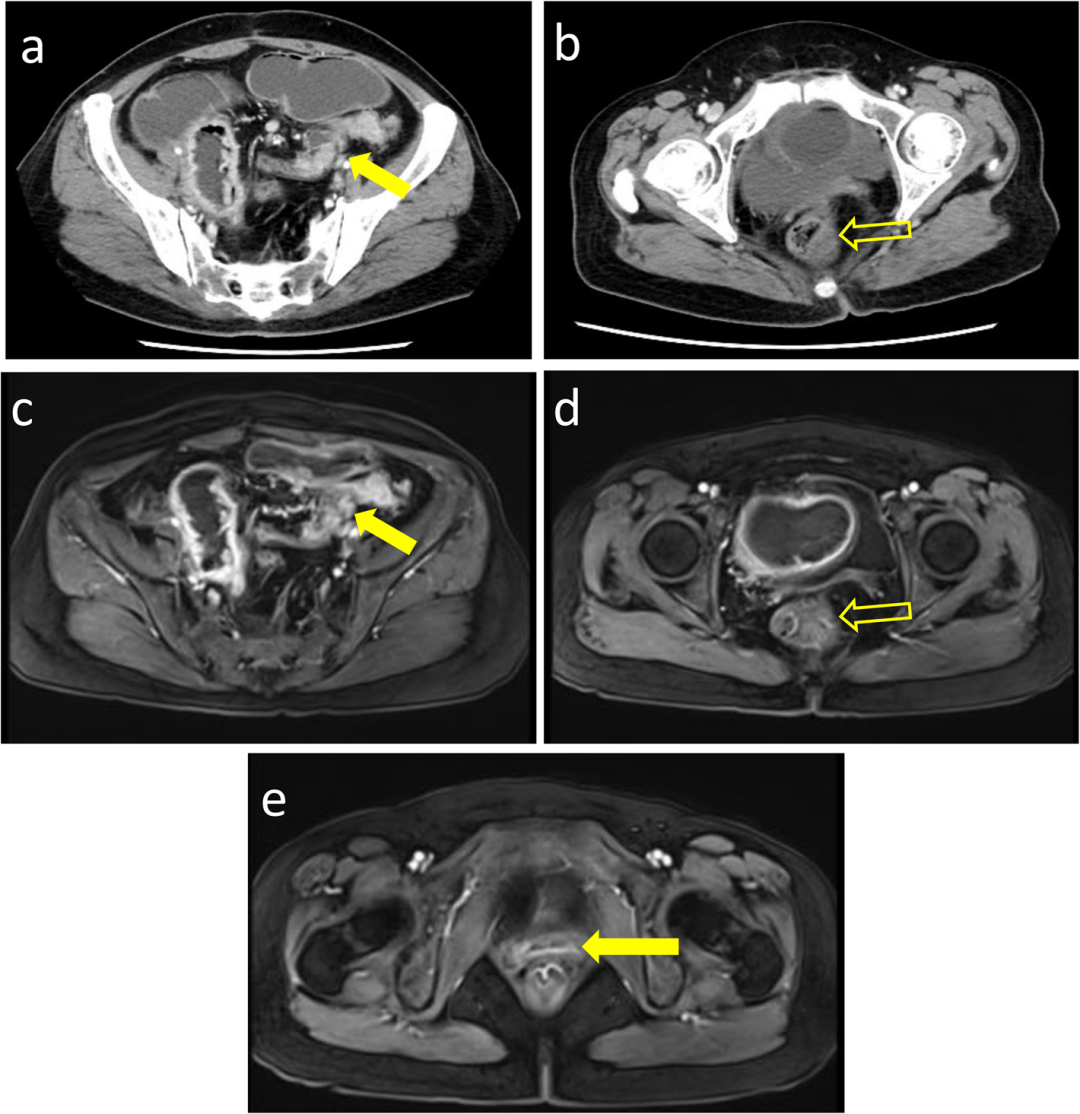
Abdominal computed tomography (CT) scan. CT scan revealed lower intestinal obstruction with a thickened bowel wall in the terminal ileum (*solid yellow arrow* in (a) and upper sigmoid colon (*feint yellow arrow* in (b). Abdominal magnetic resonance (MR) revealed a thickened bowel wall in the terminal ileum (*solid yellow arrow* in (c) and upper sigmoid colon (*feint yellow arrow* in d). MR imaging also showed an unremarkable uterus stump (*solid yellow arrow* in e)
